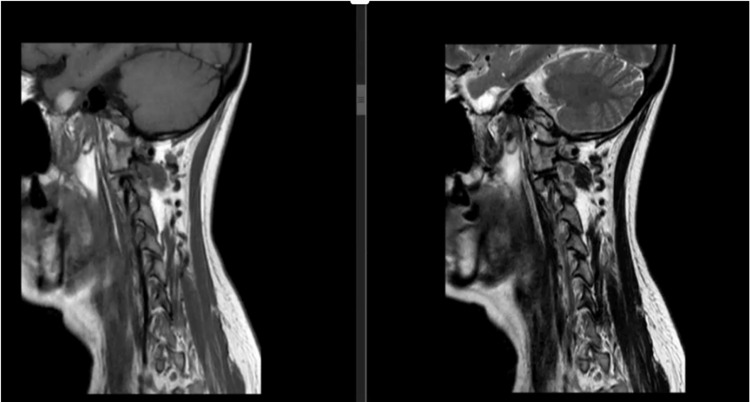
Cervical MRI displaying straightening of the normal cervical lordosis and intrinsically normal cervical cord without abnormal areas of enhancement. Right: T2-weighted. Left: FLAIR: fluid-attenuated inversion recovery.
